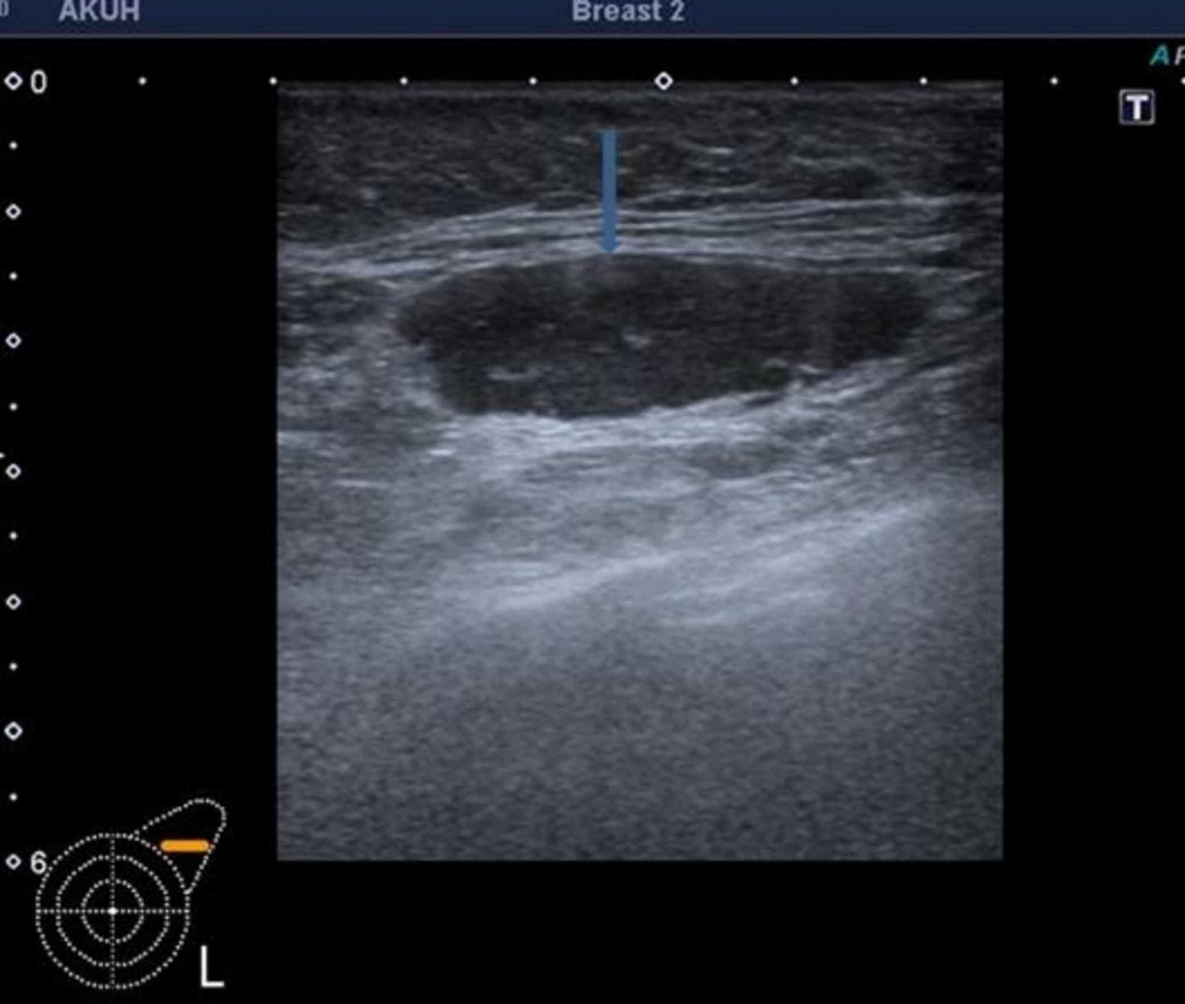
Ultrasound showing an enlarged left axillary node with loss of fatty hilum and thickened cortex (arrow).
